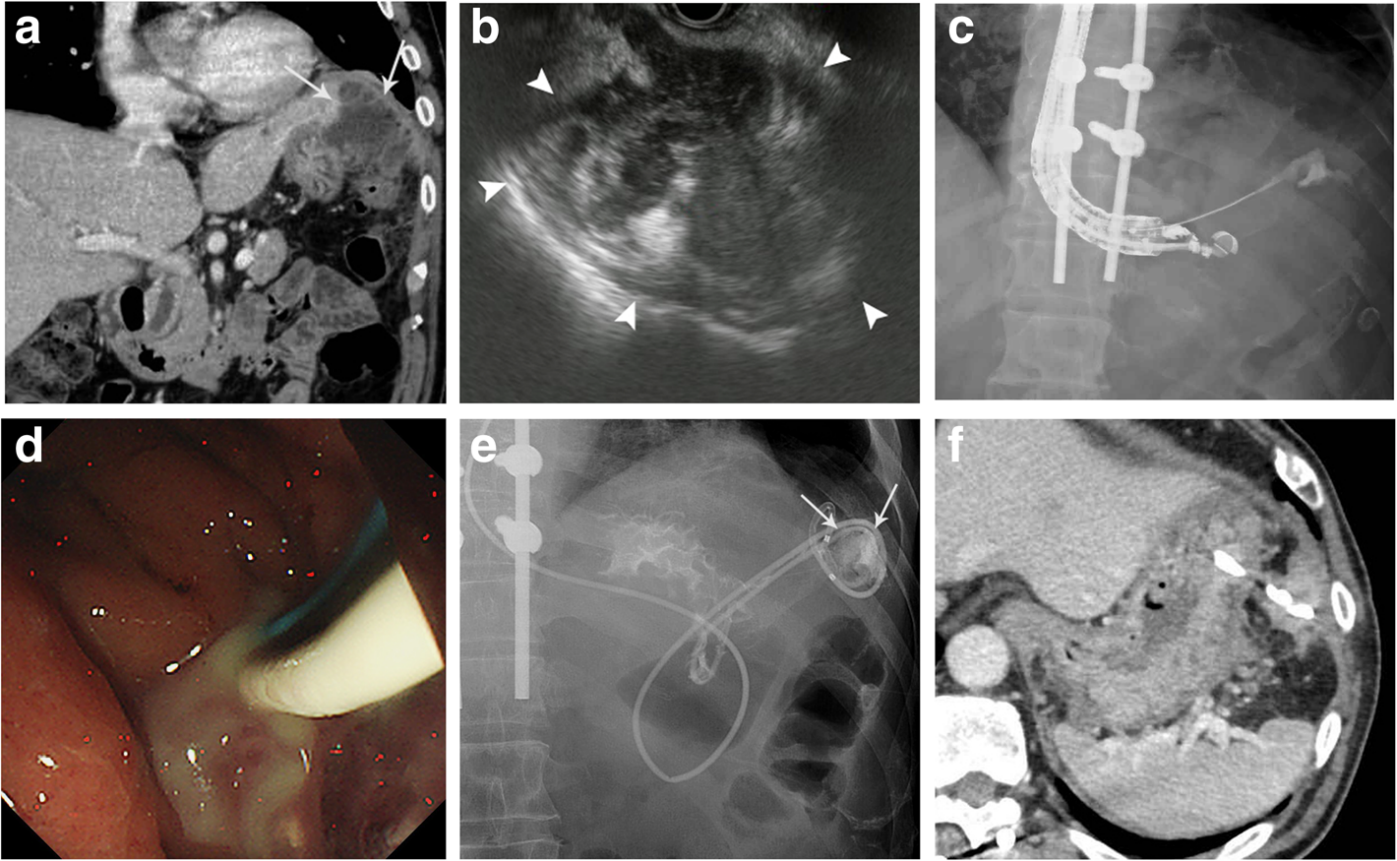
a Contrast-enhanced computed tomography (CT) shows fluid collection (arrows) in the left subphrenic area adjacent to the fornix of the stomach. b Endoscopic ultrasound shows irregular fluid collection (arrowheads). c Fluoroscopic image shows a 19-gauge needle inserted into the abscess cavity. d Endoscopic image shows much viscous pus extruding into the stomach through the stents. e Fluoroscopic image with contrast medium enhancement via the naso-abscess external catheter shows shrinkage of the abscess cavity (arrows) and no leakage from the anastomosis of the transected colon. f CT reveals that the drainage stent and that the drained abscess cavity became shrank
